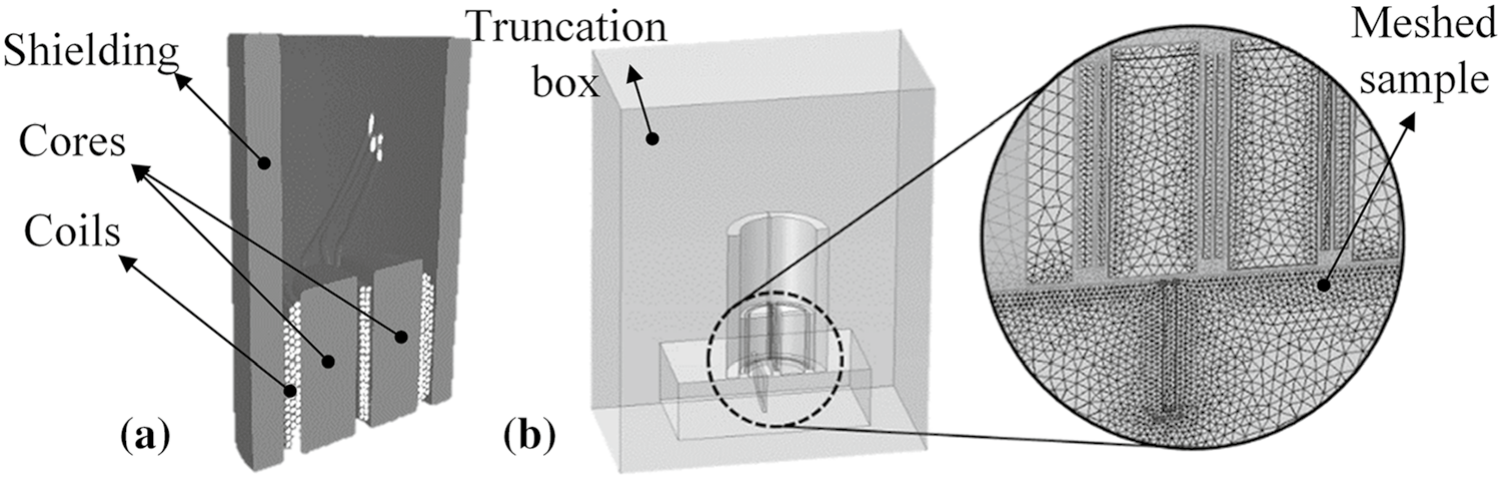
a Cut in half 3D reconstruction of X-Ray tomography images of the split-D probe, b the probe and sample assembly model prepared within COMSOL Multiphysics^®^ showing a small meshed region in close proximity to the notch geometry
